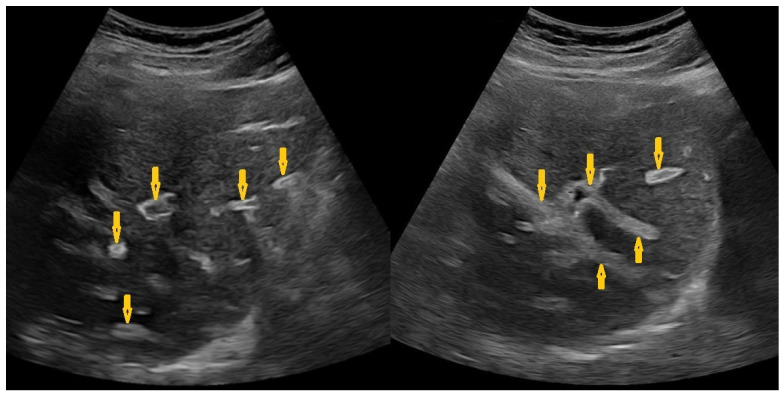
Selected sections of ultrasonography of a 79-year-old man’s liver show irregular borders, coarse texture, periportal fibrosis (arrows), and signs of portal hypertension, including mild splenomegaly. Picture of chronic liver disease with portal hypertension as a result of chronic Schistosomiasis.
